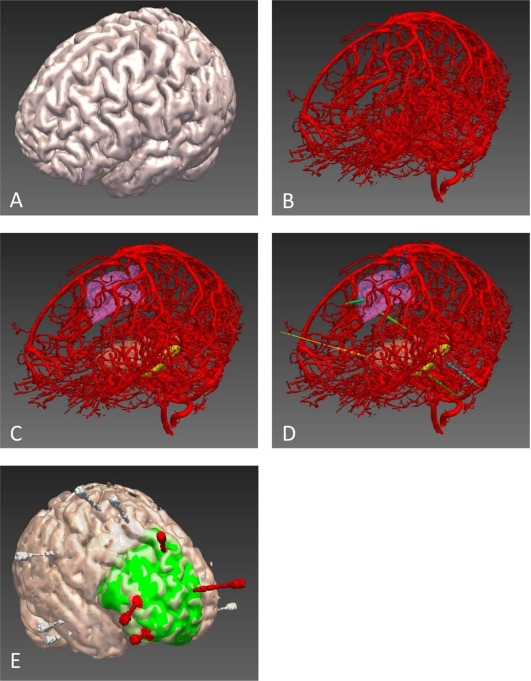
(A) Three‐dimensional cortical model. (B) Vascular segmentation from digital subtraction angiogram following left internal carotid and vertebral injections. (C) Vascular segmentation with automated parcellation of anatomical regions of interest (ROIs), including supplementary motor cortex, anterior insula, and hippocampus. (D) Automated electrode trajectory placement targeting predefined anatomical ROIs. Not all of the implemented electrode trajectories or target ROIs are shown in this image. (E) Postimplantation reconstruction of a different patient to that shown in A–D. Bolt and electrode contact points are segmented from postimplantation computed tomography and overlaid on cortical model. Electrodes with contacts implicated at seizure onset are shown in red, whereas those not involved at seizure onset are shown in white. Planned resection volume to include electrodes at seizure onset is shown in green. For clarity, the bolts are displayed, not the individual electrode contact points.
